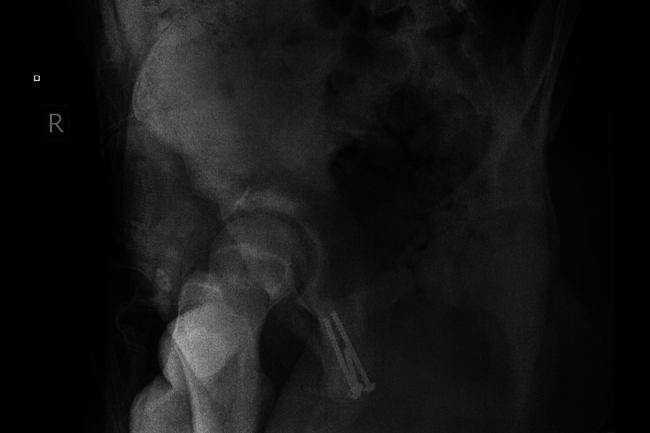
Judet view radiograph of the right hip one month postoperatively showing the fixation in place.
